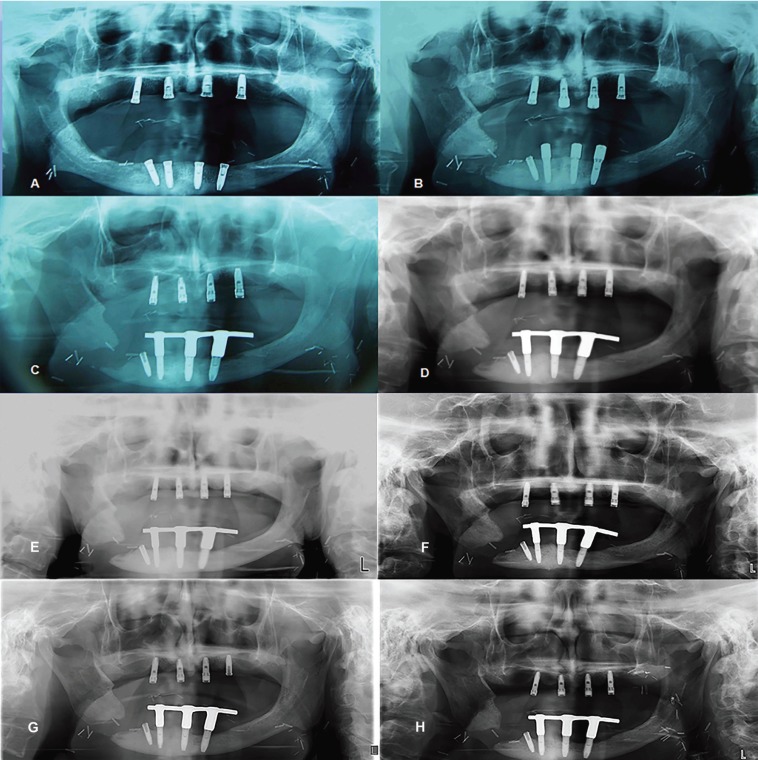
X-ray controls. The mandibular fracture remains separated without an evidence of bone callus formation, over the time. A) December 2008 B) August 2009 C) October 2010 D) October 2011 E) May 2012 F) March 2013 G) December 2015 H) August 2018.
